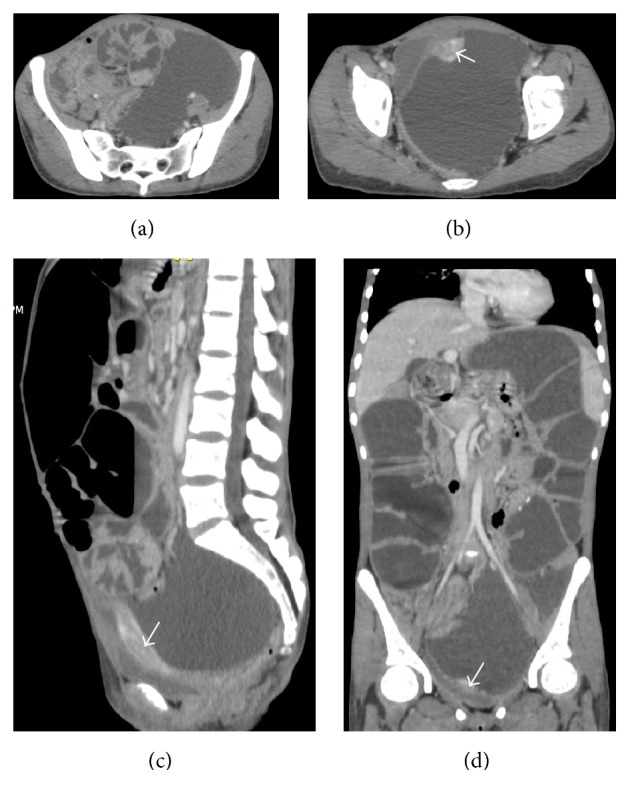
Contrast enhanced abdominopelvic CT-scan (portal phase). Axial (a, b), sagittal (c), and frontal (d) images demonstrated a large cystic pelvic mass extended to the left iliac fossa, with enhanced regular thin wall. There is mass effect on the uterus (arrow) and distended small bowel loops.
